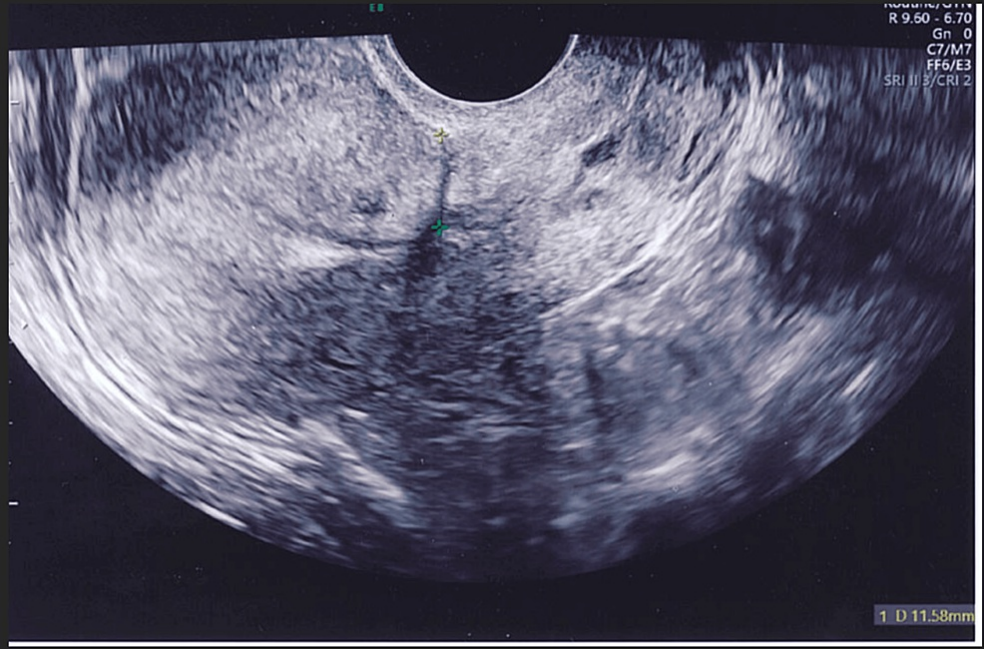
A patient who underwent parallel-layer closure. Postpartum ultrasound shows an anteverted uterus and a visible cesarean scar but no isthmocele (residual myometrial thickness of 11.5 mm).
